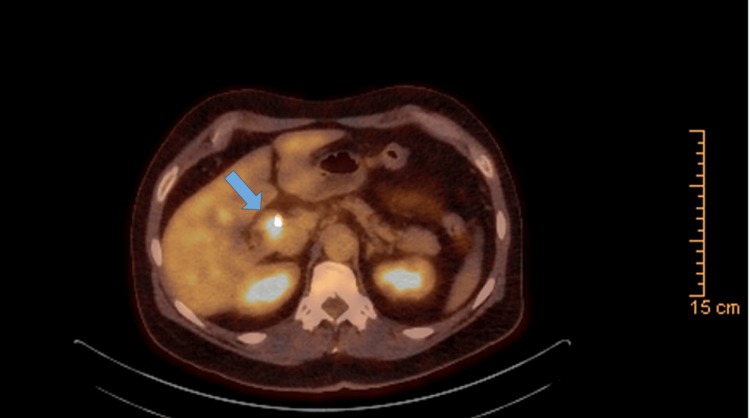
PET-CT shows intense FDG uptake along biliary stent (blue arrow). Biopsy of CBD stricture showing IPMN in a similar location. The FDG avid lesion within the gallbladder was suspicious for malignancy. PET-CT, positron emission tomography-computed tomography; FDG, fluorodeoxyglucose; CBD, common bile duct; IPMN, intraductal papillary mucinous neoplasm
